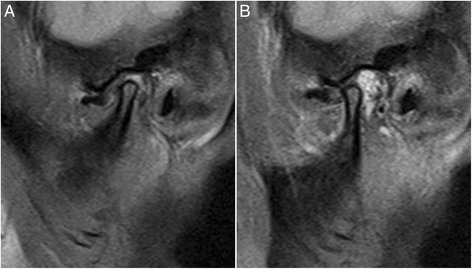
MRI Sagittal view showing disc displacement without reduction; A. Closed mouth; B. Open mouth.
